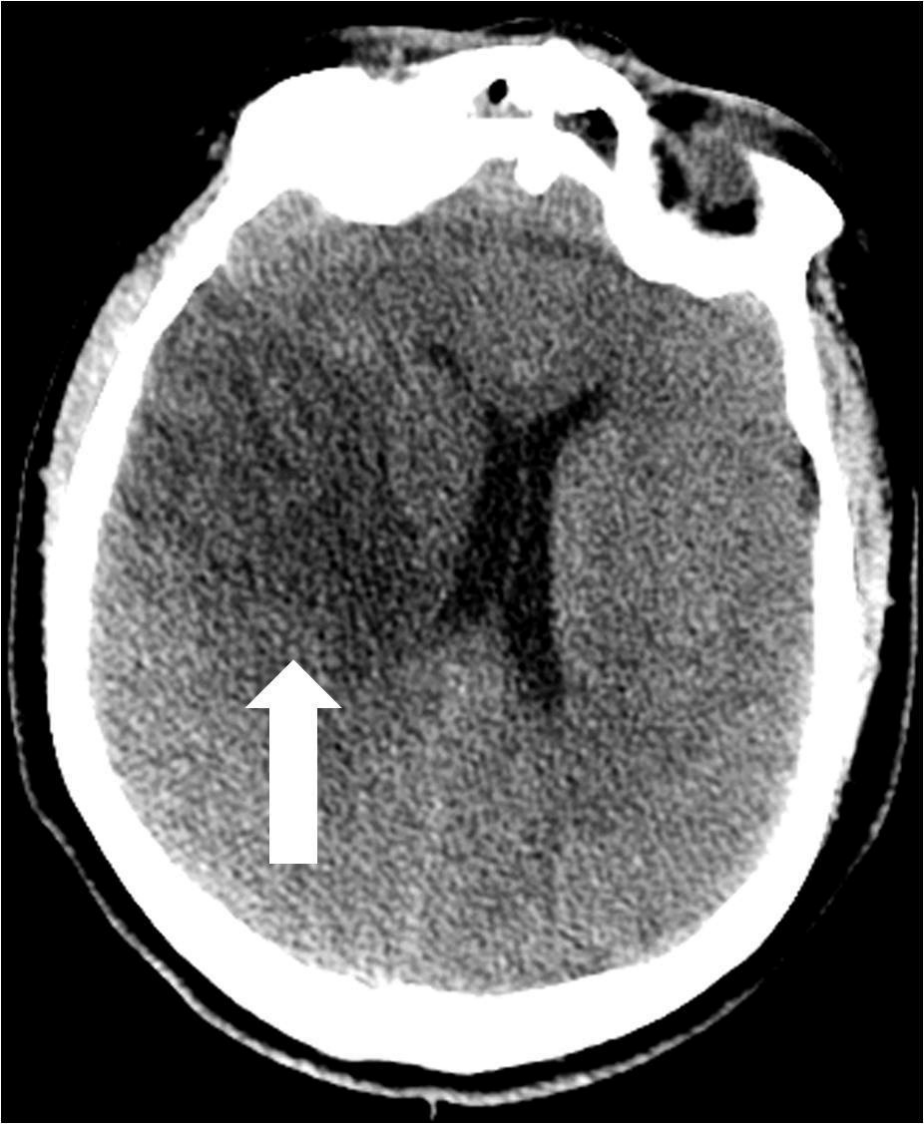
Computed tomography of the head performed five days after the traumatic event reveals the expected evolution of the right middle cerebral artery distribution infarct (arrow) with mild mass effect and right-to-left midline shift.
